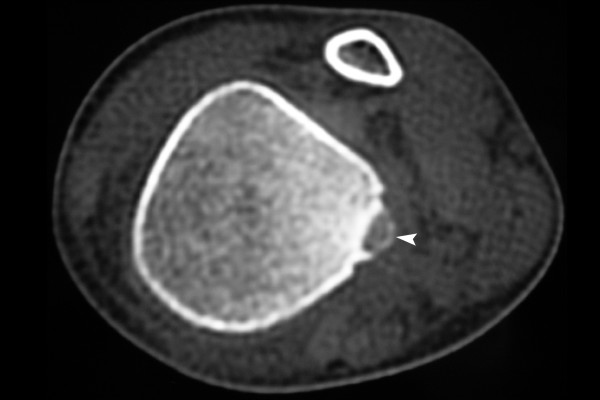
CT scan showing the same lesion as in the X-ray, with doubtful calcification inside(white arrow head).
